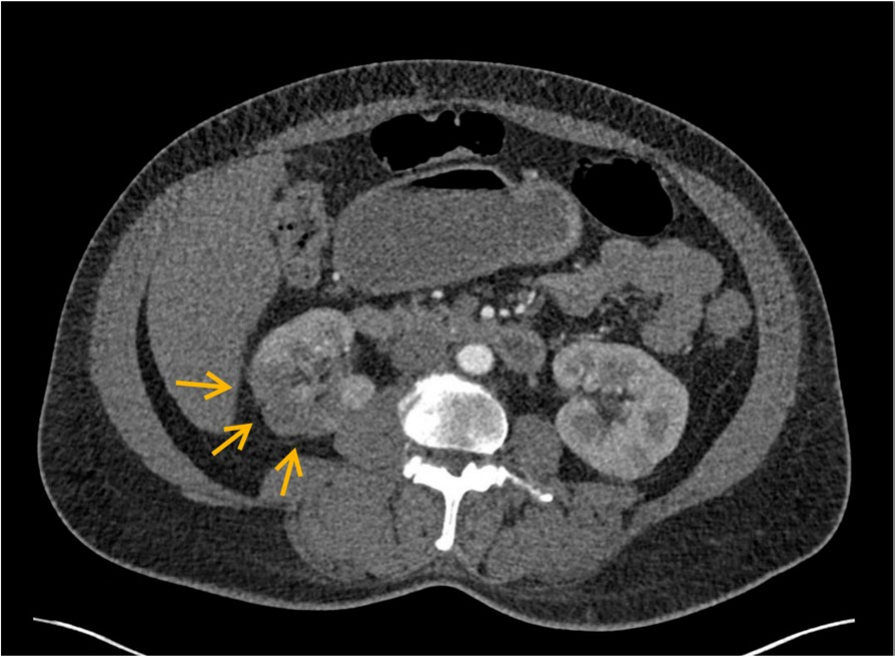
CT performed about 1 month after revealing hypodense lesions and cortical thinning of the mid and upper pole of right kidney consistent with scarring due to previous ischemic insult (arrows)
